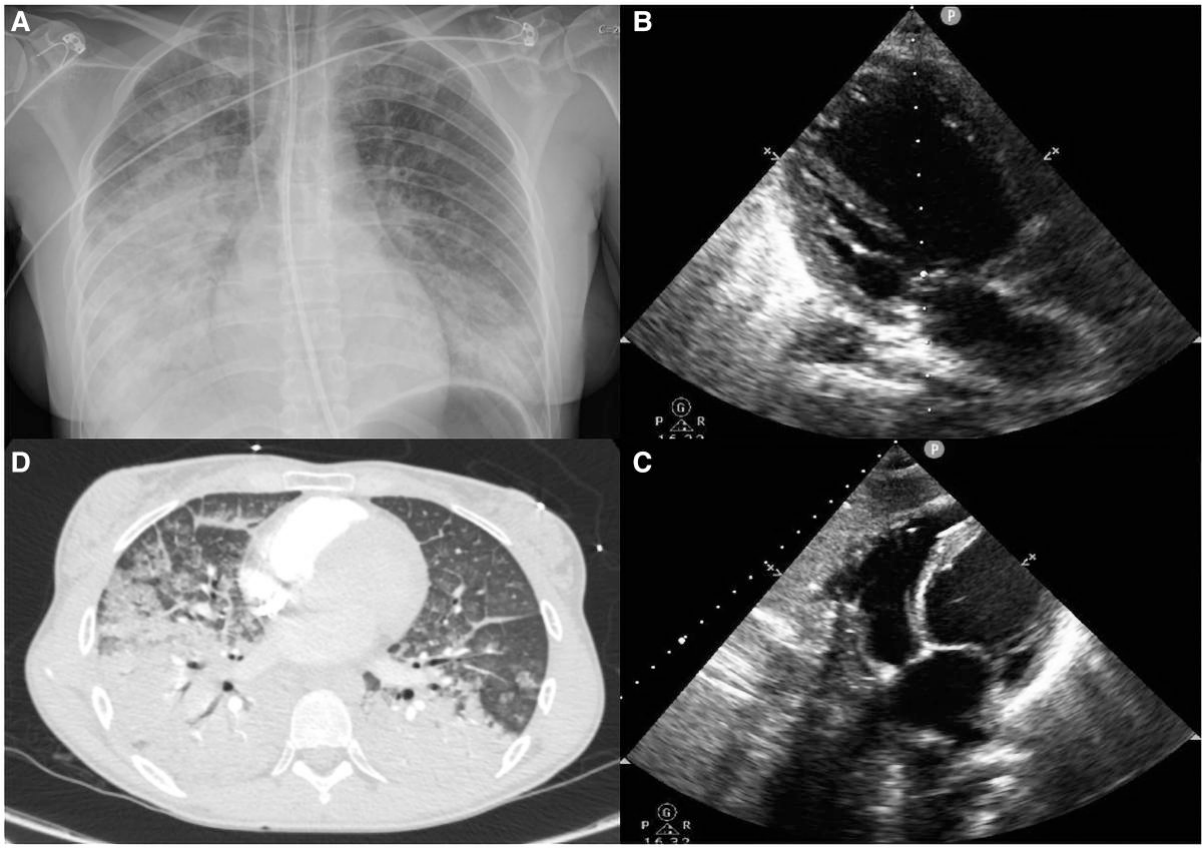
Bilateral pulmonary infiltrates, predominantly in the perihilar and basal regions, are visible on chest X-ray (*A*) and chest computed tomography (*D*), consistent with acute pulmonary oedema. Transthoracic echocardiography images showing a dilated left ventricle with severe systolic dysfunction (*B* and *C*).
